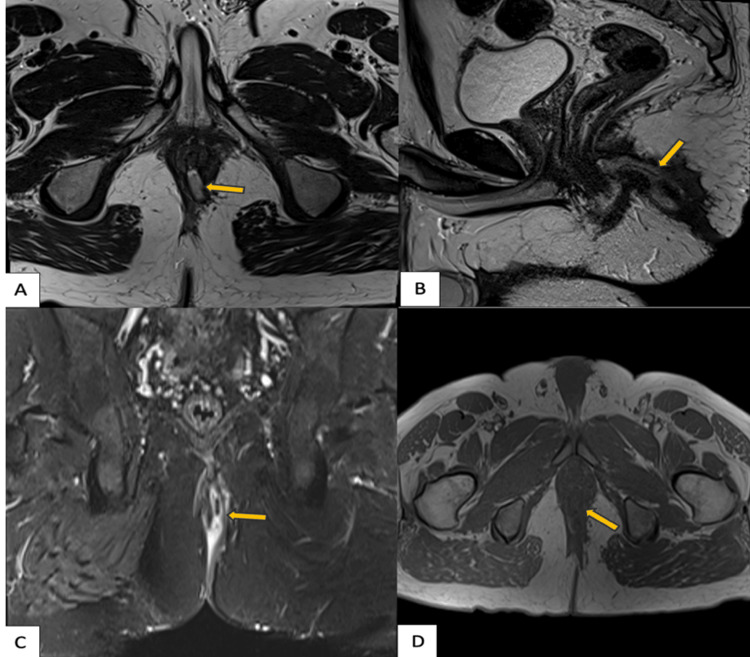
MRI fistulogram demonstrating a St. James grade 3 perianal fistula MRI: magnetic resonance imaging; STIR: short tau inversion recovery Axial (A), sagittal (B), T2-weighted, coronal STIR (C), and axial (D) T1-weighted images reveal a transsphincteric fistulous tract (orange arrows) extending through both the internal and external sphincters into the ischioanal fossa. Mild surrounding inflammation is noted without associated abscess or secondary extensions (C)
